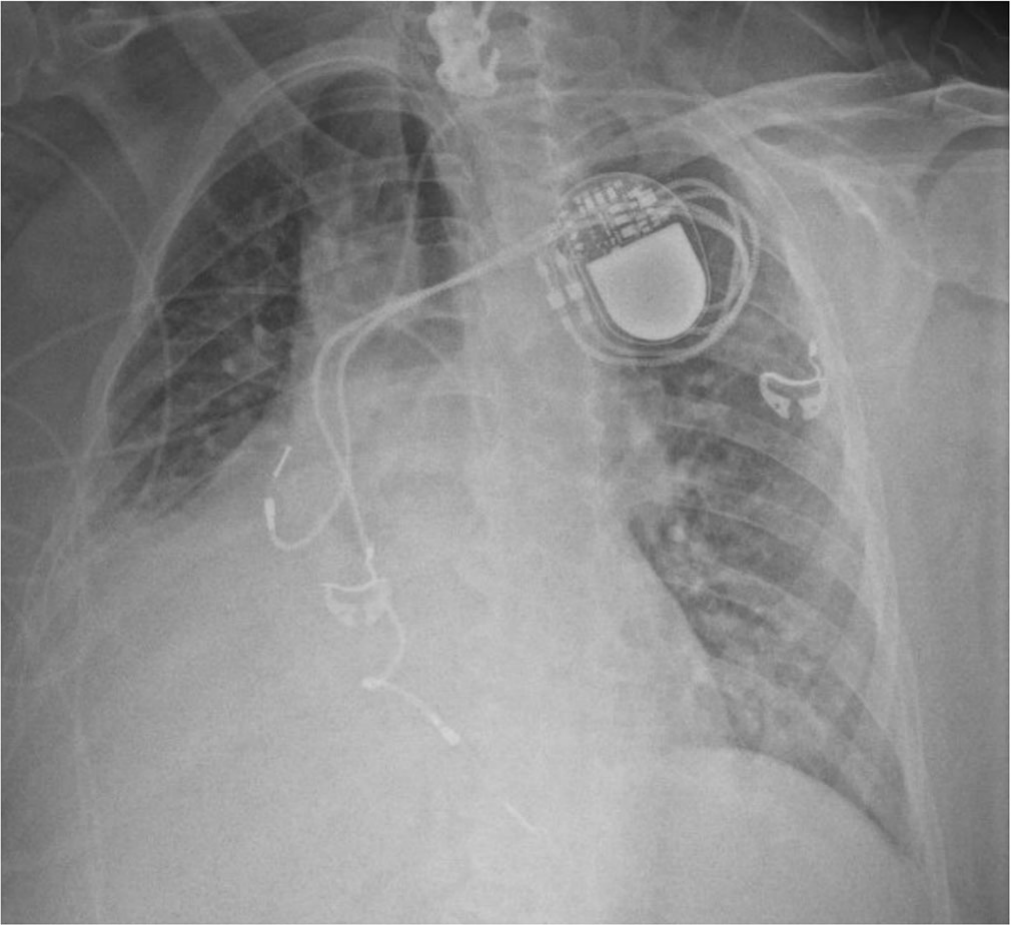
Baseline chest radiograph demonstrating the patient's dual‐chamber cardiac device in situ, before any lead extraction attempts.
